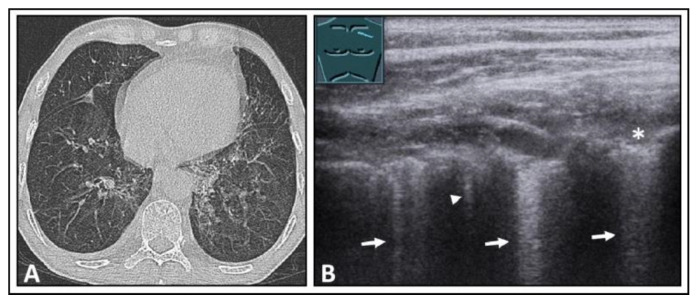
A patient with secondary acute myeloid leukemia following allogeneic bone marrow transplantation, with graft-versus-host disease (GVHD) of the intestines and increasing dyspnea. (A) The computed tomography scan shows mosaic-like ground-glass opacities on both sides, emphasized in the upper lobes, as well as peribronchial consolidations, consistent with acute GVHD of the lung. (B) On lung ultrasound, multiple B-lines (arrows) and a comet-tail artifact (arrowhead) are seen in the area of the upper lobe, with a rough, interrupted pleural reflection line (*) as seen with a focal interstitial pattern.
